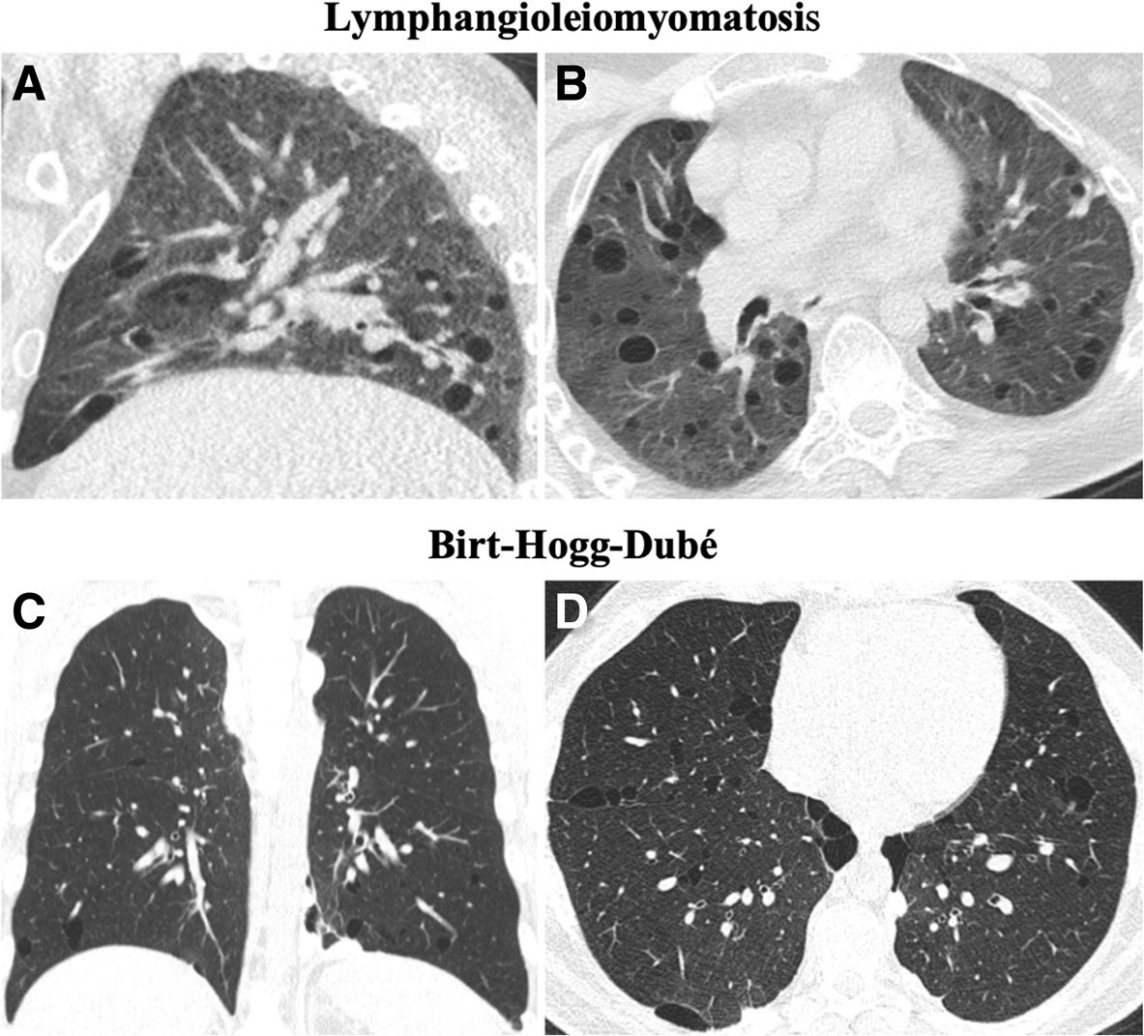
Differential diagnosis of PLCH. a Sagittal and b axial CT images show a 50-year-old woman with LAM. There are multiple rounded-shaped cysts, with a relatively uniform distribution with no zonal predilection and affecting the lung bases. c Coronal and d axial CT images depict multiple cysts with a lower zone distribution, in a 35-year-old male with Birt-Hogg-Dubé syndrome. The patient had a history of recurrent pneumothoraces
